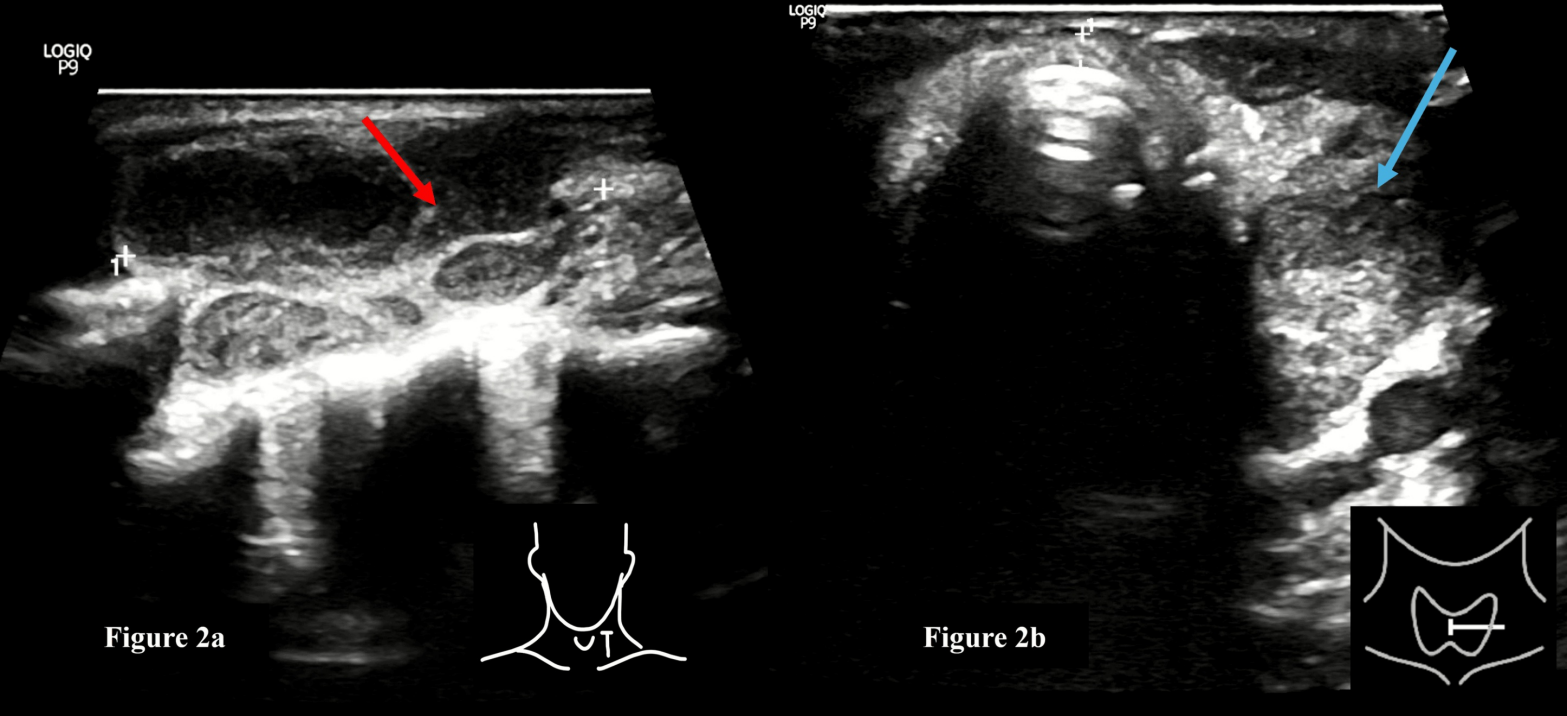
Ultrasonography of the neck a: Ultrasonography of the neck region on the left side (sagittal section) shows multiple conglomerate necrotic lymph nodes (red arrow); b: Ultrasonography of the neck region (transverse section) shows a well-defined, thick-walled cystic area with echogenic content (blue arrow) with thick internal septations noted involving the left lobe of the thyroid.
